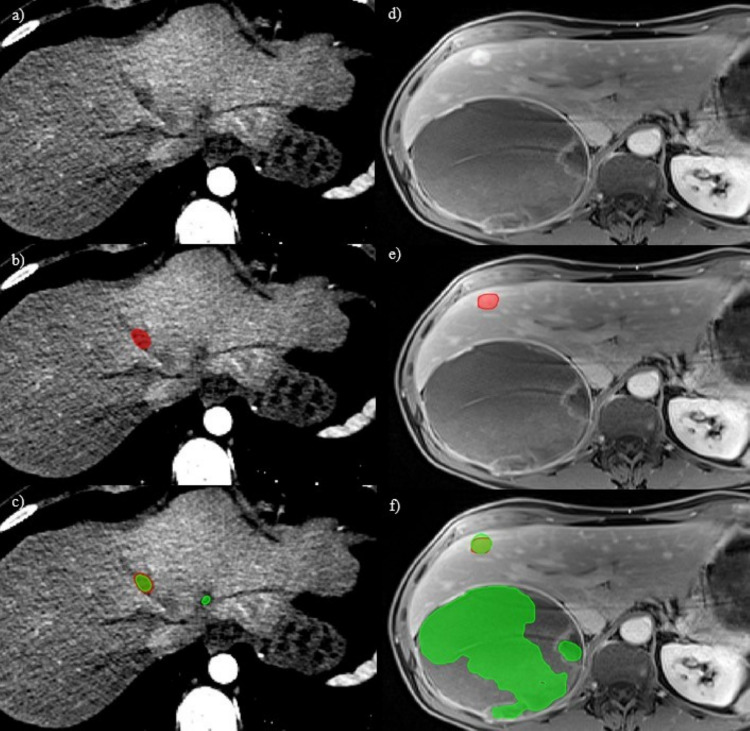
Cross-sectional examples in CT and MRI demonstrating both correct and incorrect model segmentation outcomes. a–c CT modality: a Original CT slice; b ground-truth annotation of a hemangioma in liver segment IV (red); and c model segmentation overlay, which correctly identifies the hemangioma but also produces a false positive by misclassifying a blood vessel as a lesion (green). d–f MRI modality: d Original MRI slice; e ground-truth annotation of a hemangioma in liver segment V (red); and f model segmentation overlay, which again captures the true lesion but incorrectly identifies a kidney tumor as a false positive (green). These examples demonstrate both the strength of the model in detecting true hepatic lesions across modalities and the challenge of avoiding false positives, particularly when nearby vessels or extrahepatic structures mimic lesion characteristics
